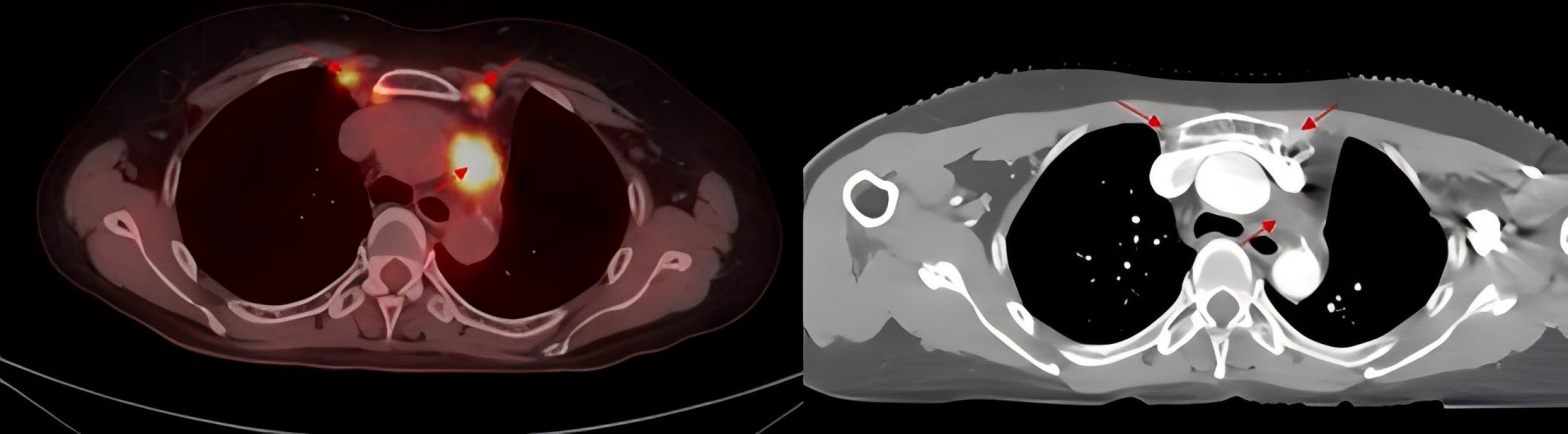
PET/CT imaging shows enlarged bilateral internal mammary and para-aortic lymph nodes and enhanced CT before thoracic radiotherapy.
